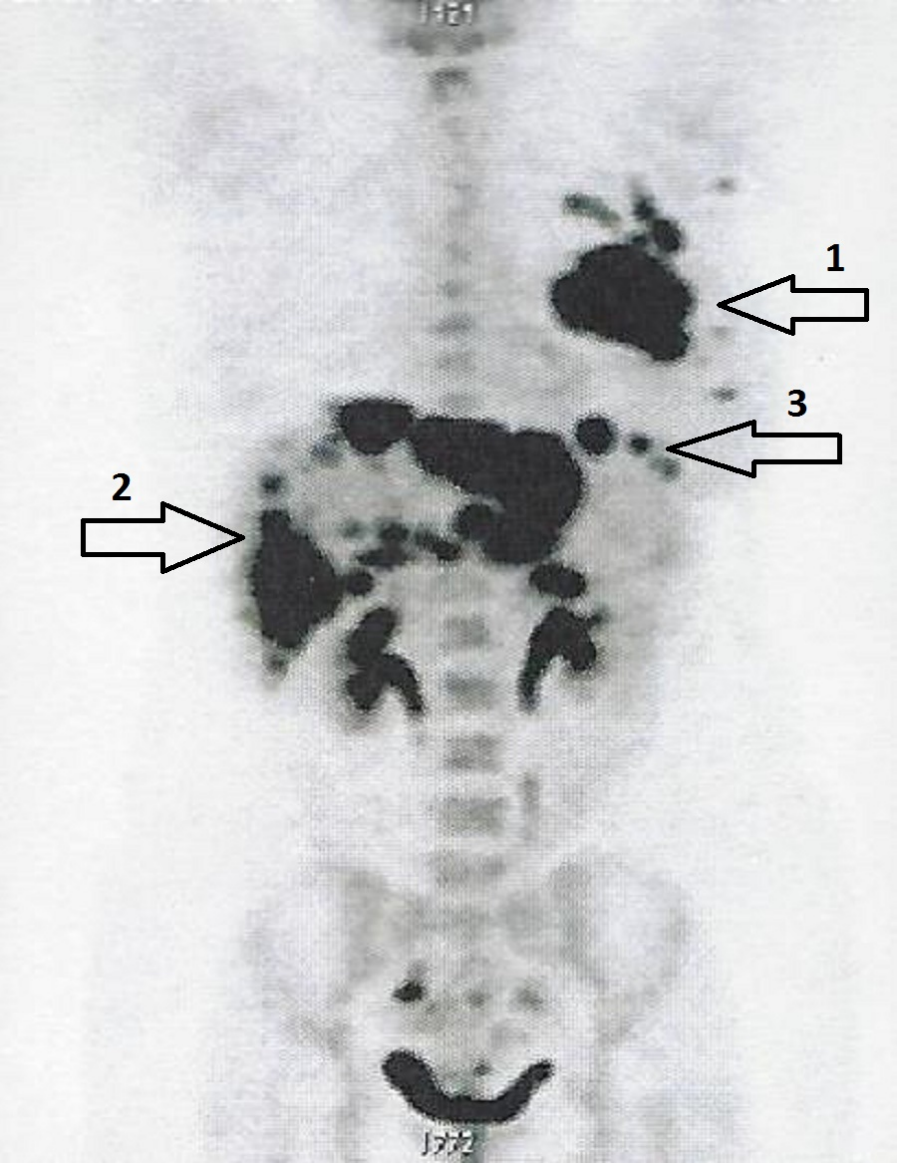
Whole body (18F)-FDG-PET-CT scan showing a 77 mm x 55 mm primary tumor in the left breast (arrow 1), multiple widespread liver masses (arrow 2), and an upper left nodular abdominal lesion (arrow 3). The metastases are so widespread, the use of arrows is nearly insufficient. Abbreviations: CT, computed tomography; FDG, fluorodeoxyglucose; PET, positron emission tomography.
